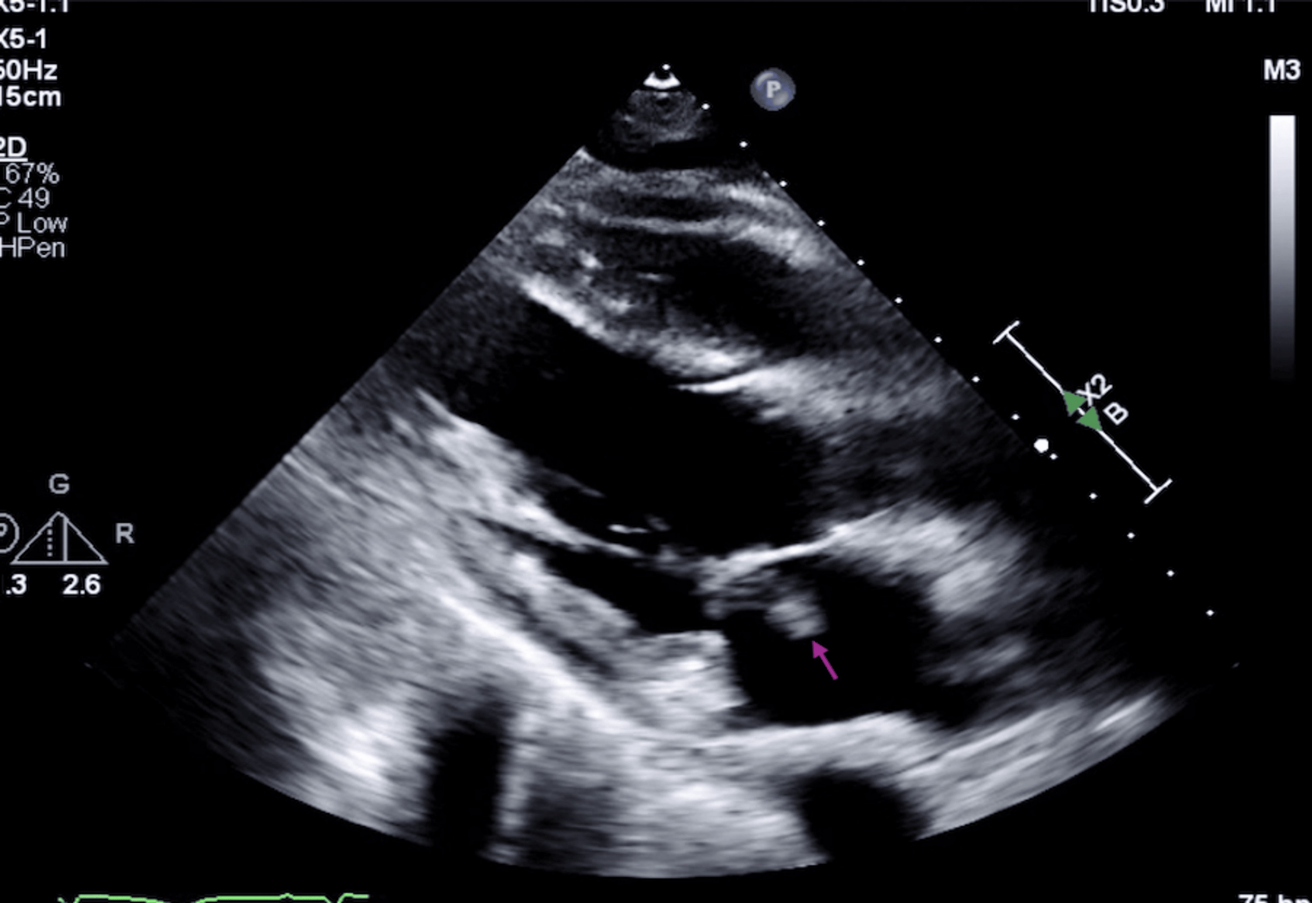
Parasternal long-axis transthoracic echocardiographic view demonstrating a large mitral valve vegetation (pink arrow)
